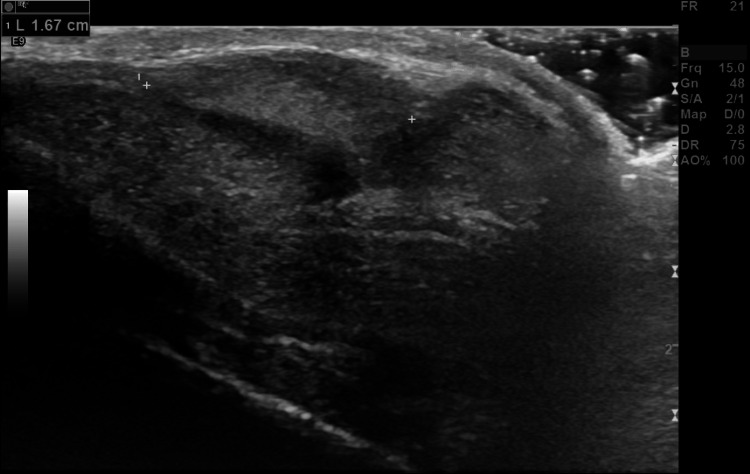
Ultrasound demonstrating a 1.67 cm slightly hyperechoic soft tissue mass overlying the medial aspect of the right corpus cavernosum. The mass is adjacent to the cavernosal tissue and measures 1.6 × 0.8 × 1.4 cm.
